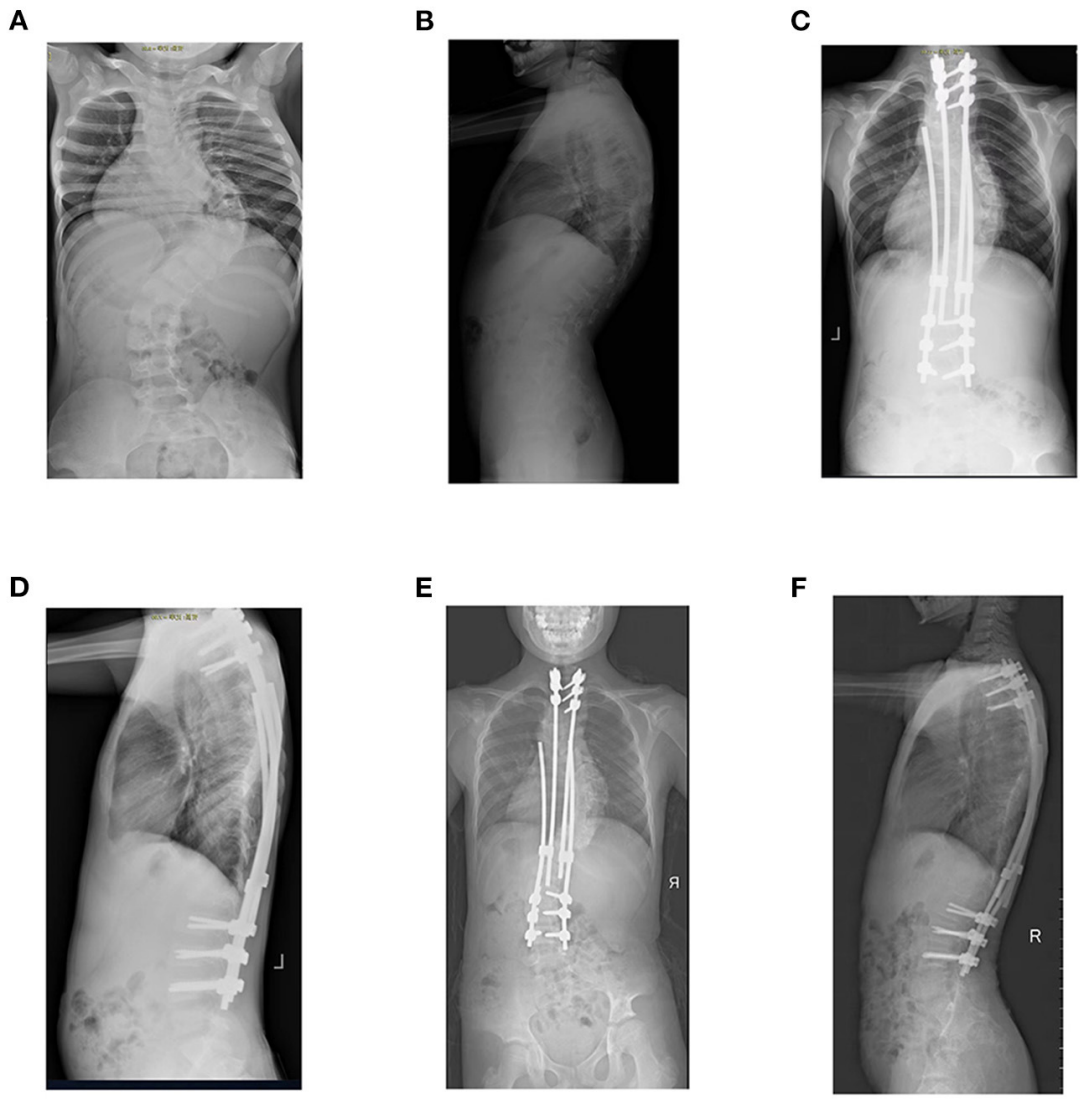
Boy aged 7 years and 8 months diagnosed with kyphotic early-onset scoliosis. (A,B) preoperative anteroposterior and lateral radiographs of the spine indicate coronal lateral bending of 91° and sagittal thoracic kyphosis of 63°. (C,D) X-ray re-examination shows satisfactory correction of kyphosis after growing-rod implantation. (E,F) X-ray re-examination at the 38th month of follow-up (the 4th strutting surgery) showed a satisfactory correction effect and an increase in spinal height.
